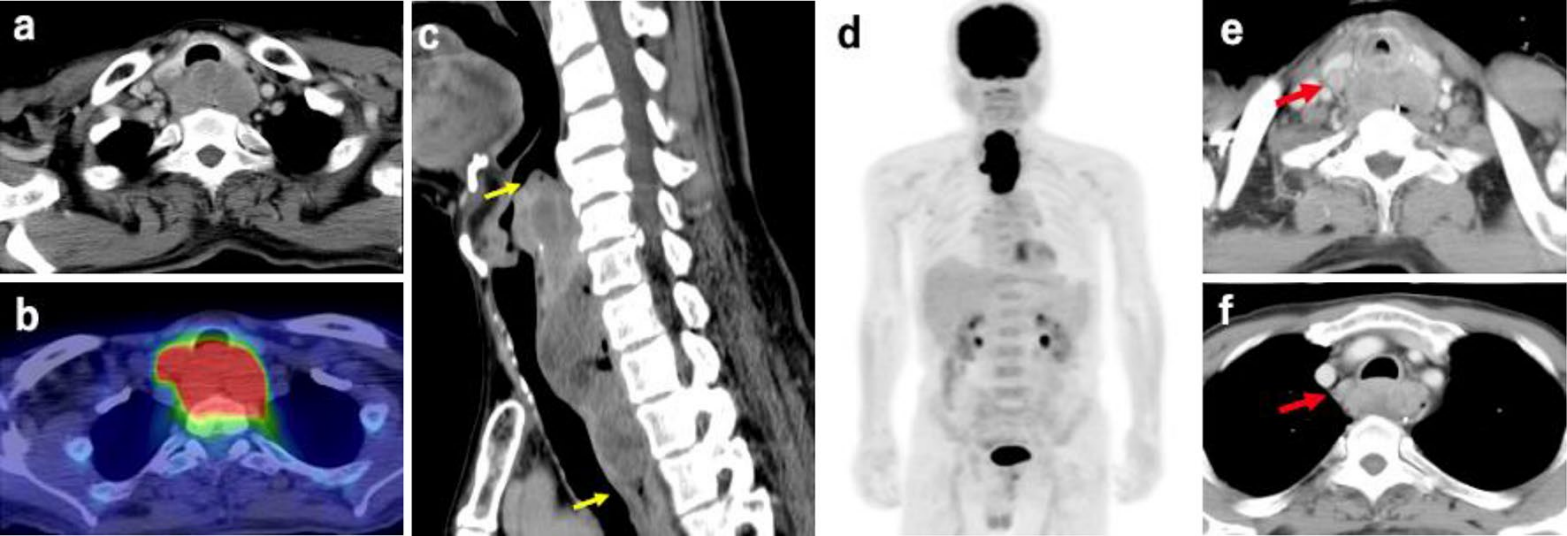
Contrast-enhanced CT and PET-CT findings. The tumor with entire circumferential wall thickening (a) with high 18F-fluorodeoxyglucose accumulation (b), which extended from the epiglottis to the upper thoracic esophagus (delineated by the yellow arrows) (c), invaded the trachea in the hypopharyngeal cervical esophagus, and no evidence of distant lymph node metastasis and other distant metastasis was found (d). There were, however, several metastases to regional lymph nodes (red arrows) (e, f) (colour figure online)
